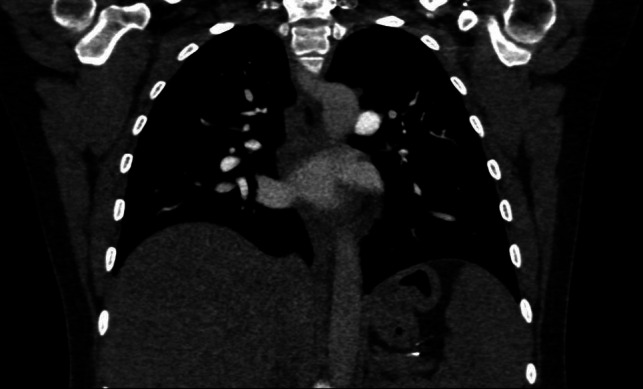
Chest CT scan, showing gastric prolapse
